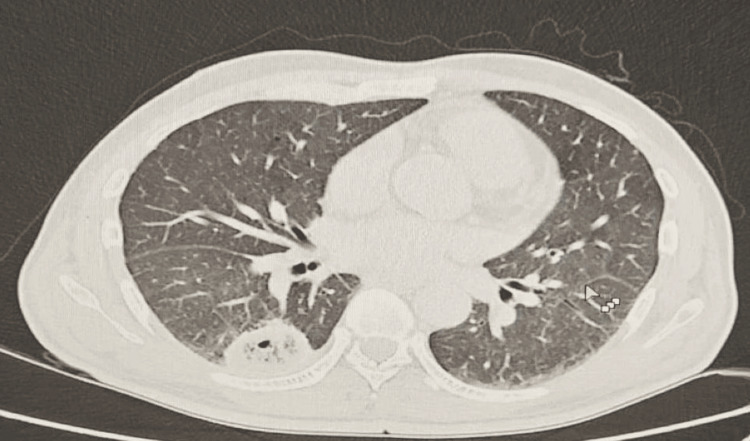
Contrast-enhanced CT chest showed multiple bilateral pulmonary cavitary lesions, largest in the right lower lobe measuring 29 x 44 x 33 mm
